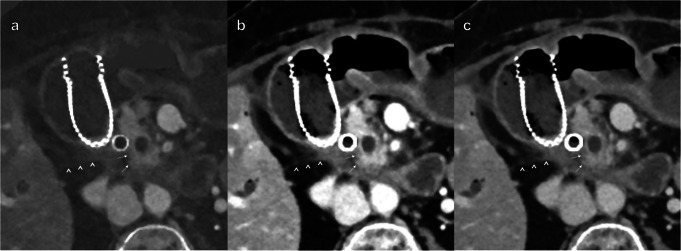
Axial contrast-enhanced abdominal CT scans in portal venous phase. Image a is MD-iodine, image b is VMI at 55 keV, and image c is VMI at 70 keV. The images are of a 79-year-old female patient with a PDAC in the head of the pancreas partially surrounding the biliary stent. The white arrows indicate the border between the tumor and normal pancreatic parenchyma. The arrowheads indicate tumoral strands extending laterally from the tumor into the retroperitoneal fat. One of the most experienced readers (Reader 1) graded MD-iodine in this case as Score 1 for the parameter lesion conspicuity (Lesion not visualized at all). The remaining image reconstructions were graded as Score 2
